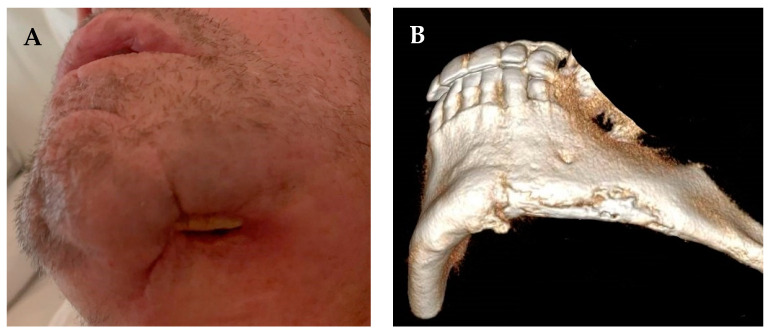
Mandibular ORN following mandibulotomy and radiotherapy for squamous cell carcinoma of the base of tongue. (A) clinical image demonstrating dehiscence of the soft tissue and exposure of necrotic bone on the inferior border of the left mandibular parasymphysis and body and (B) a 3-dimensional reconstruction of the CT demonstrating sequestration of the inferior mandibular border.
